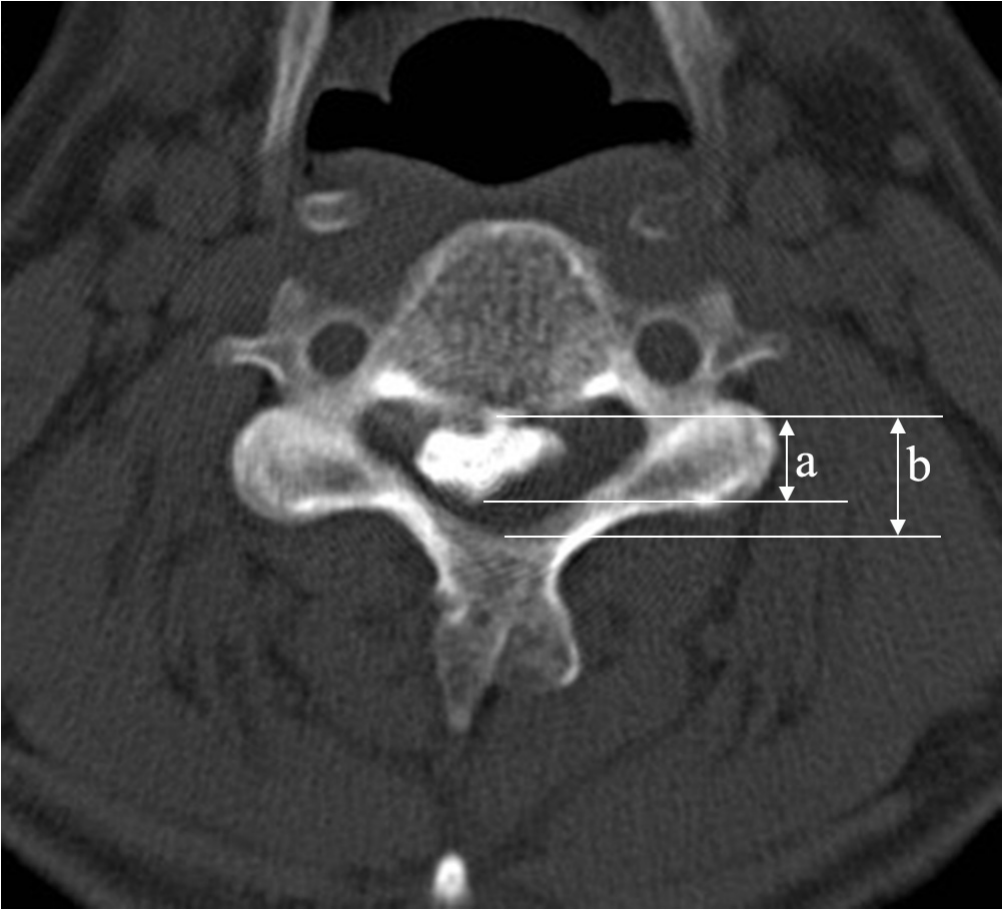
The occupying ratio of the spinal canal was defined as the ratio of the maximal ossification thickness (a) to the anterioposterior spinal canal diameter (b) on CT axial imaging.
